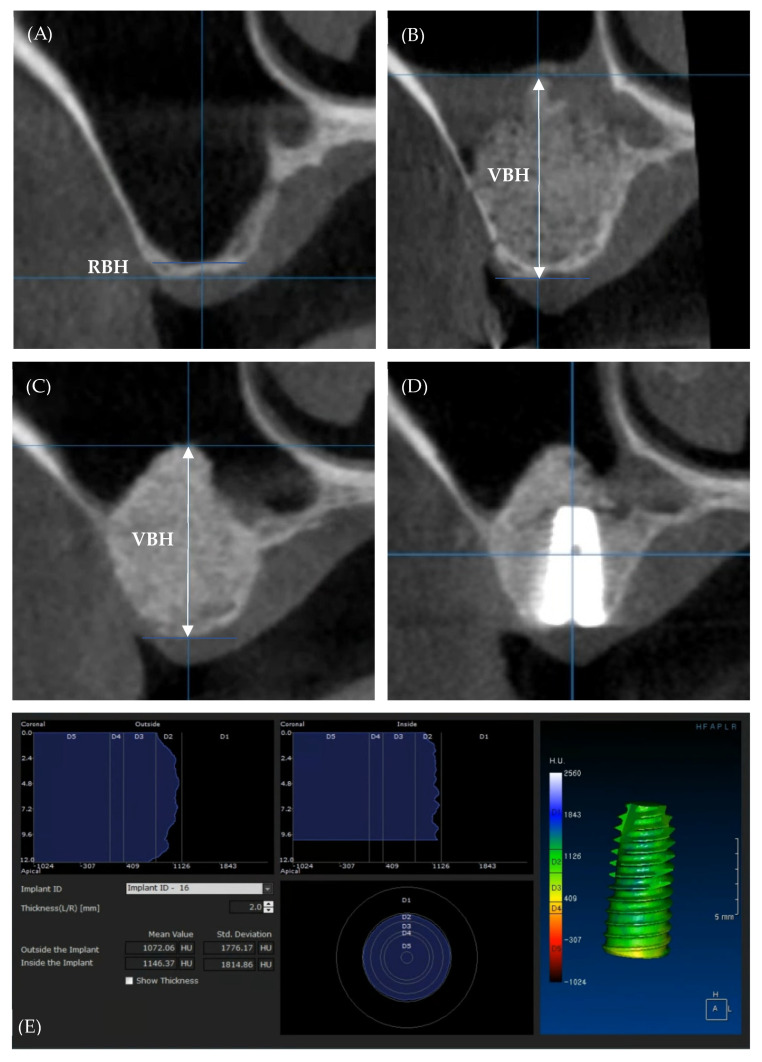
Cone−beam computed tomography images according to the implant placement site. (A) Preoperatively (T0), (B) immediately after the surgery (T1), (C) 6 months postoperatively (T2), (D) after implant placement, (E) Hounsfielid unit (HU) value measurement. RBH, residual bone height; VBH, maximum vertical bone height.
